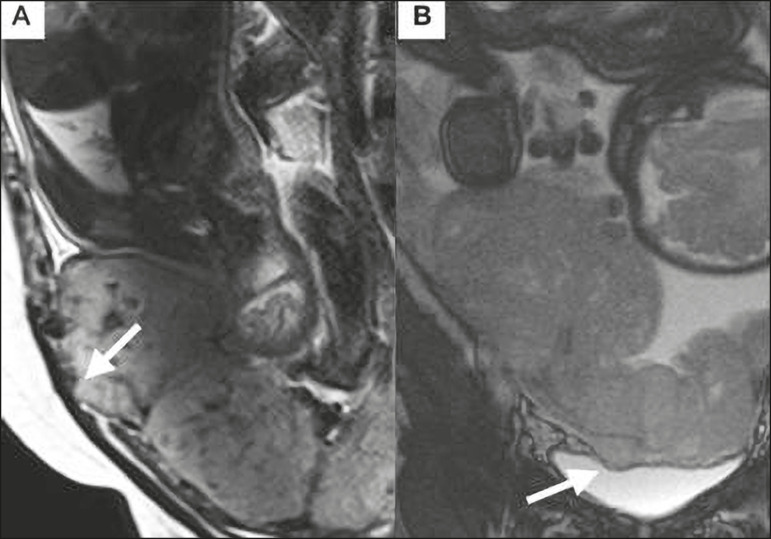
A,B: Sagittal T2-weighted MRI sequences showing a type II placental bulge (arrow in A) with a distorted uterine contour. There is also bulging of the posterior wall of the bladder caused by the placental bulge (arrow in B).
